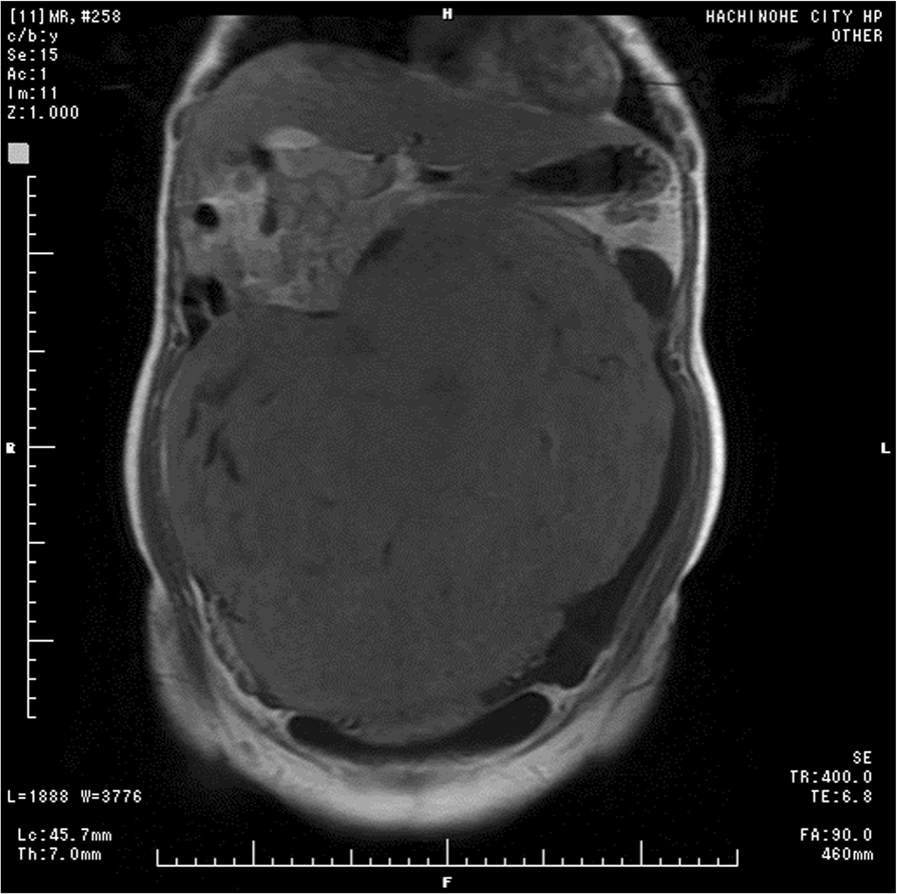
A coronal T2-weighted magnetic resonance image of the uterus
